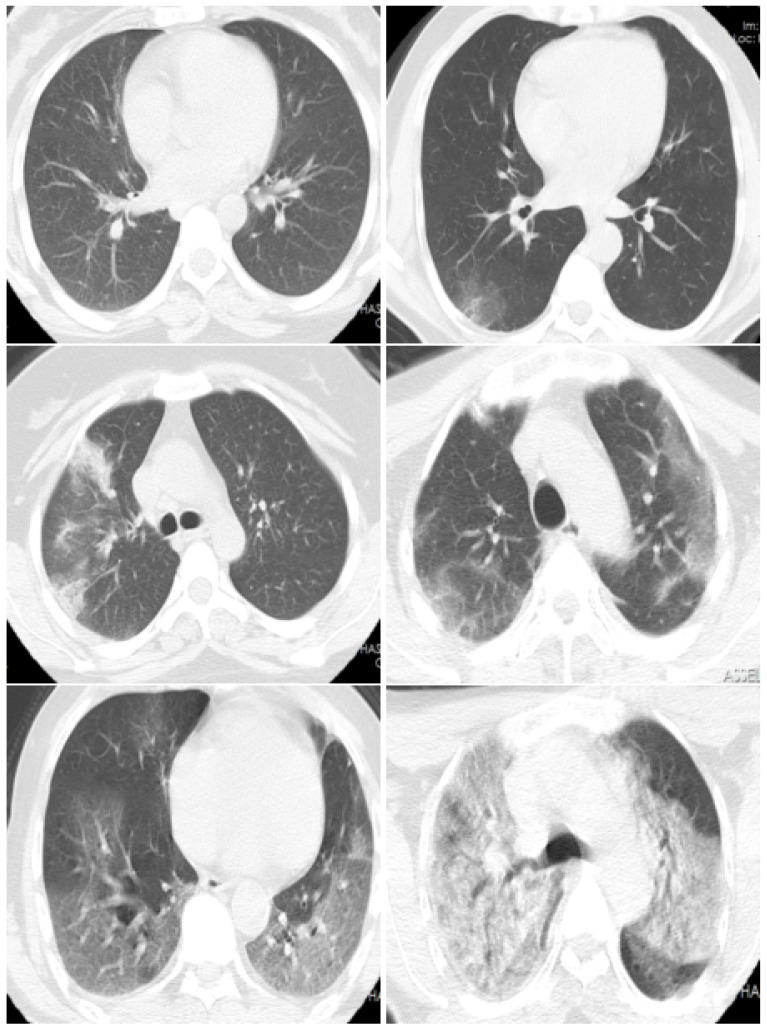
CT scan images examples which have the COVID-19 infection percentages of 0%, 5%, 18%, 40%, 70%, and 80%, respectively. These slices are classified as Normal, Minimal (<10%), Moderate (10–25%), Extent (25–50%), Severe (50–75%), and Critical (>75%), respectively.
